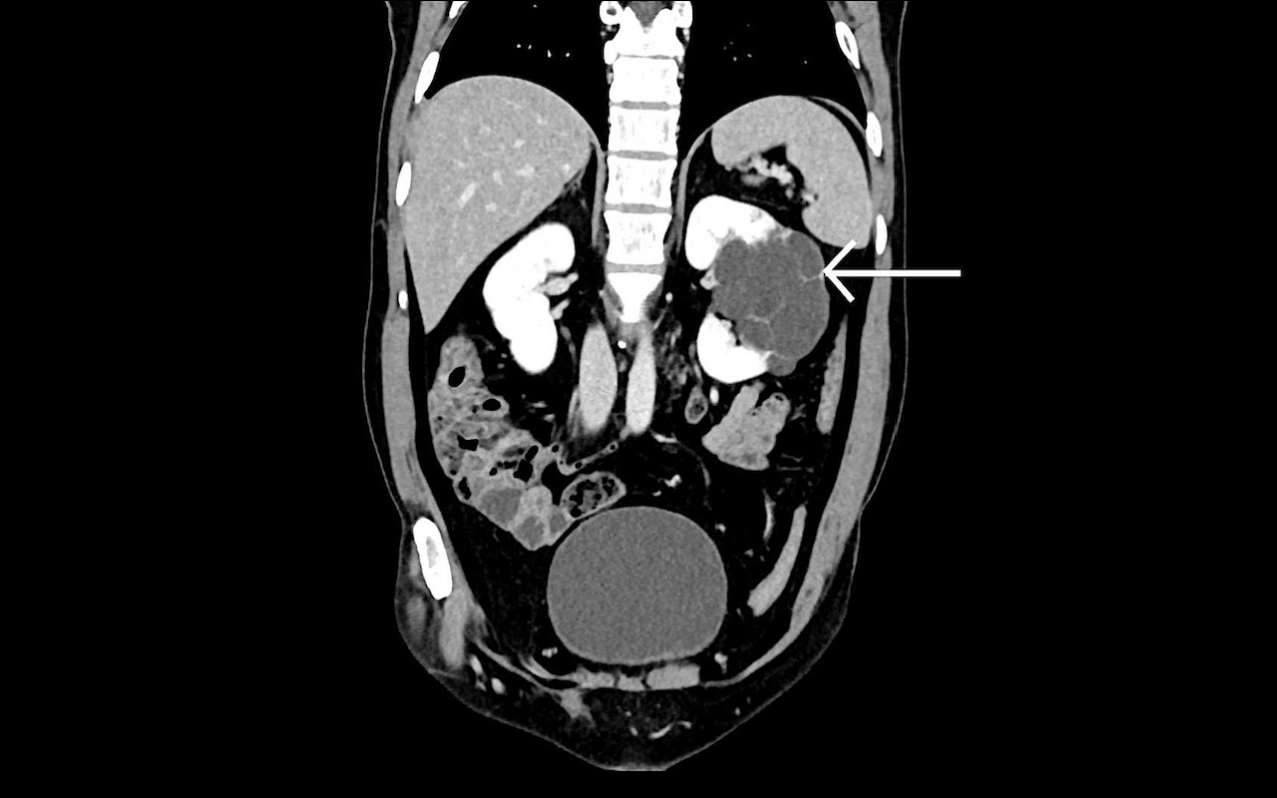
A 41-year-old female patient with complaint of left flank pain. Intravenous contrast-enhanced coronal computed tomography scan section of the affected left kidney shows multiple simple unenhanced hypodense cysts separated by contrast-enhanced thinned out residual renal parenchyma (white arrow).
